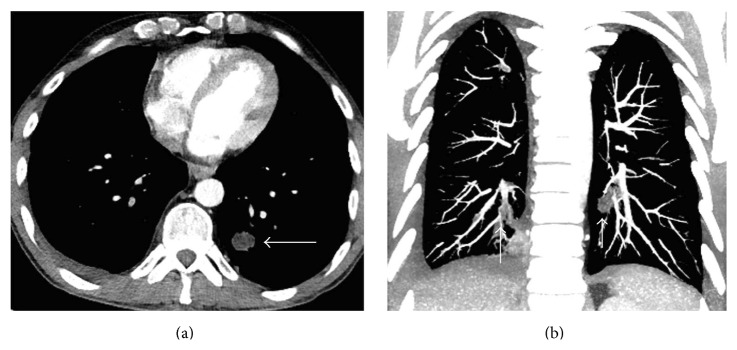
Axial contrast-enhanced CT scan (a) and coronal MIP image (b) show multiple cystic nodules in the inferior pulmonary arteries (arrows).
